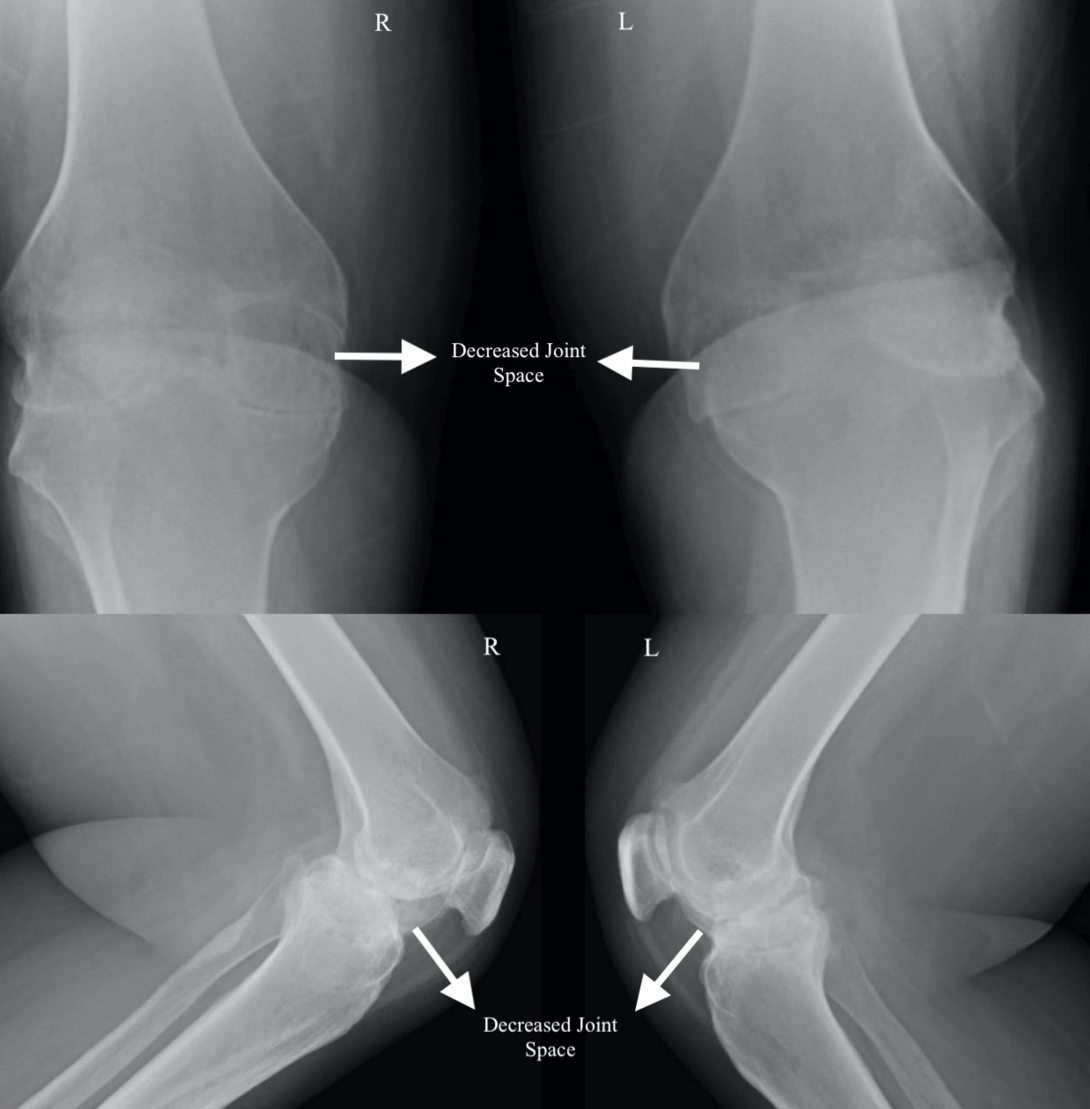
Anteroposterior and lateral X-rays showing severe osteoarthritis of both knees with decreased joint space
